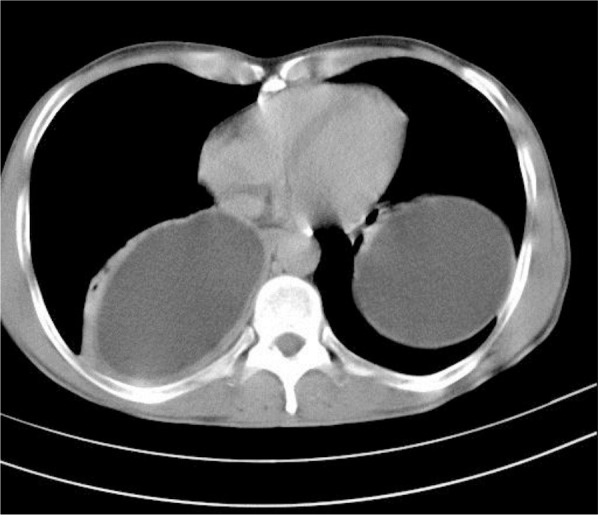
Chest CT scan shows bilateral and intact cysts.
